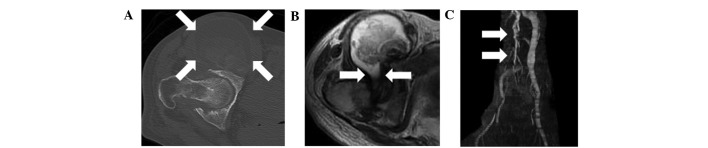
(A) Computed tomography (CT) showed an enlarged mass anterior to the right hip joint (white arrow). (B) Magnetic resonance imaging (MRI) showing an enlarged mass anterior to the right hip joint. An axial T2-weighted MRI image showed that there was a connection between the mass and the right hip joint (white arrow). (C) MRI venography demonstrated that the right femoral vein was displaced medially by the mass (white arrow).
